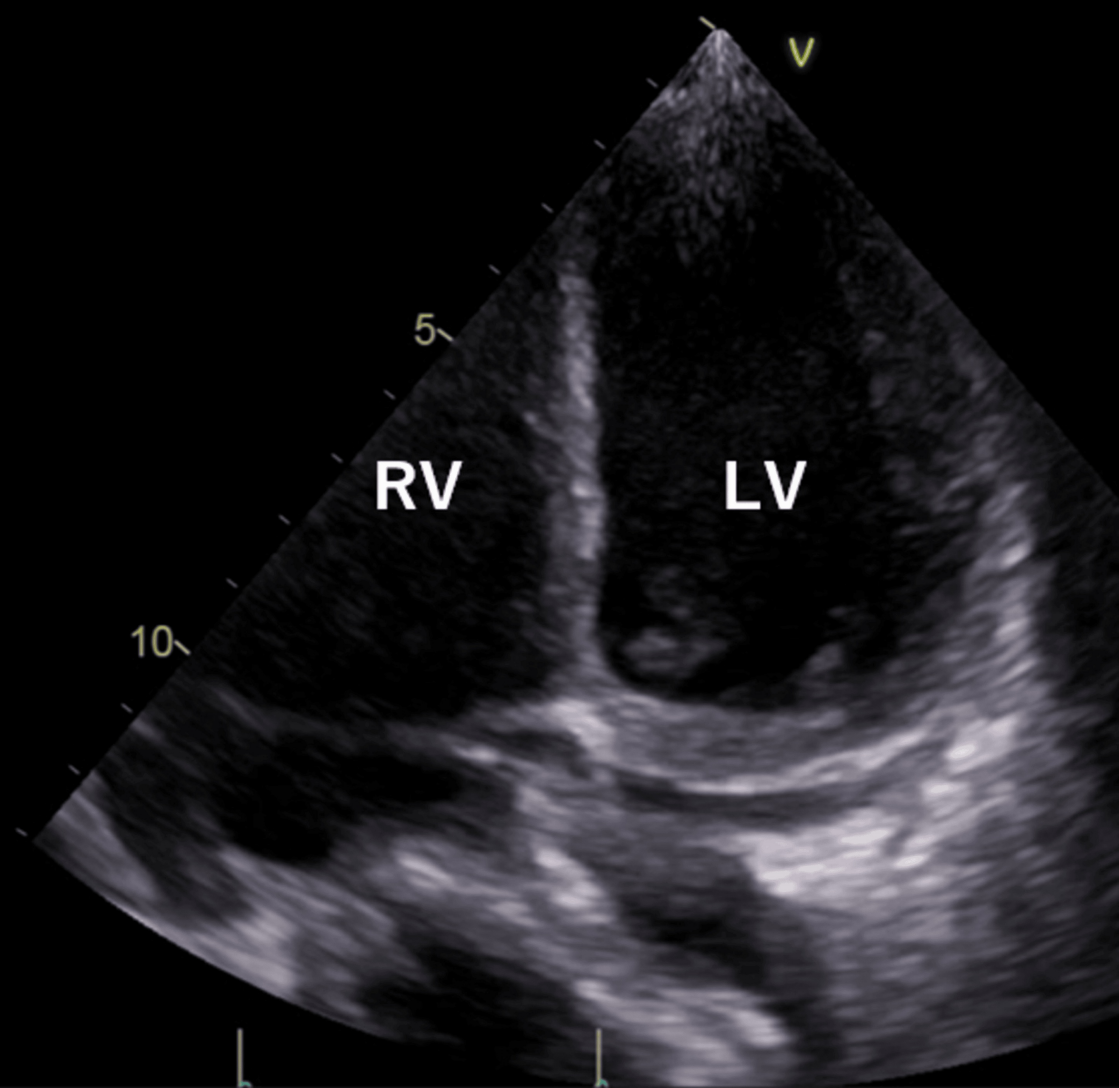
Transthoracic echocardiography. Transthoracic echocardiography on admission demonstrating diffuse left ventricular hypokinesis with a severely reduced ejection fraction (10–20%).
